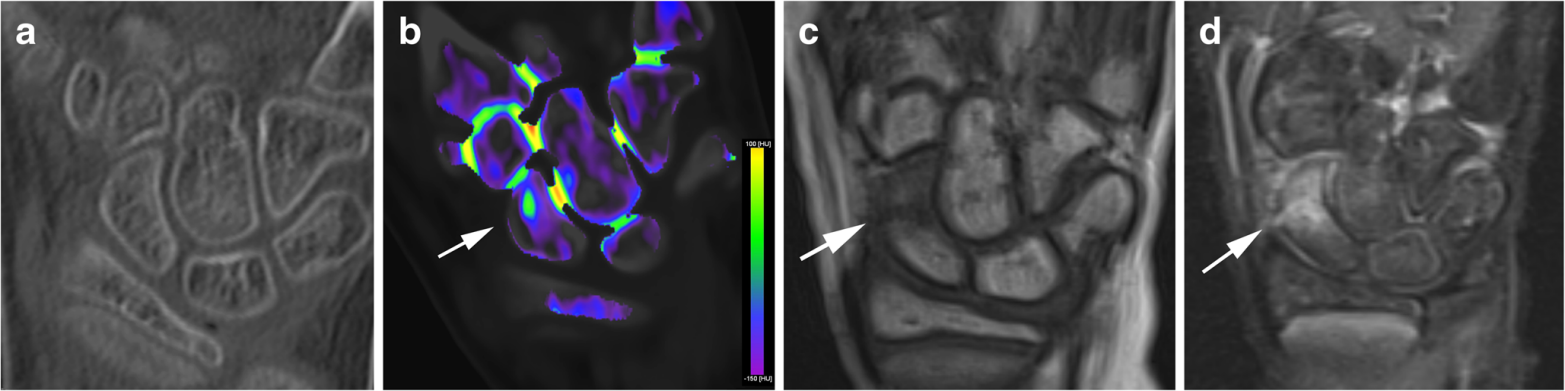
A 10-year-old female with non-displaced scaphoid fracture. CT coronal image with 2-mm slice thickness shows no fracture line (Fig. 1a). Coronal VNCa image demonstrates increased attenuation with a green color code relative to the more blue and purple normal bone, representing BME (Fig. 1b). On MRI images, the coronal T1-weighted sequence shows the low signal intensity area in the scaphoid waist (Fig. 1c) and corresponding high signal intensity on the coronal fluid-sensitive sequence (Fig. 1d), confirming the presence of BME
